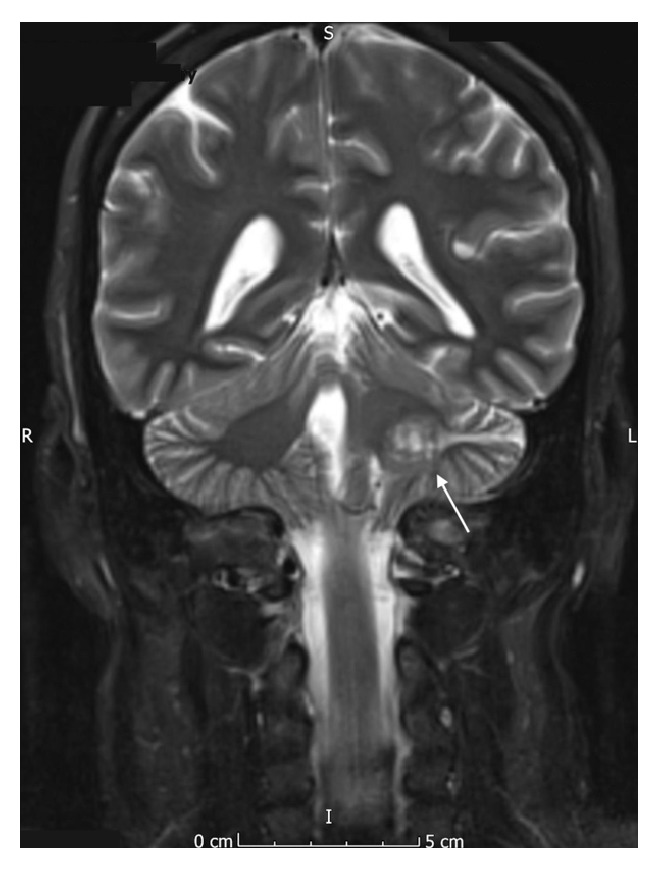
Coronal MR image (T2 weighted, fat saturated) showing the vestibular schwannoma affecting the left vestibulocochlear nerve.
